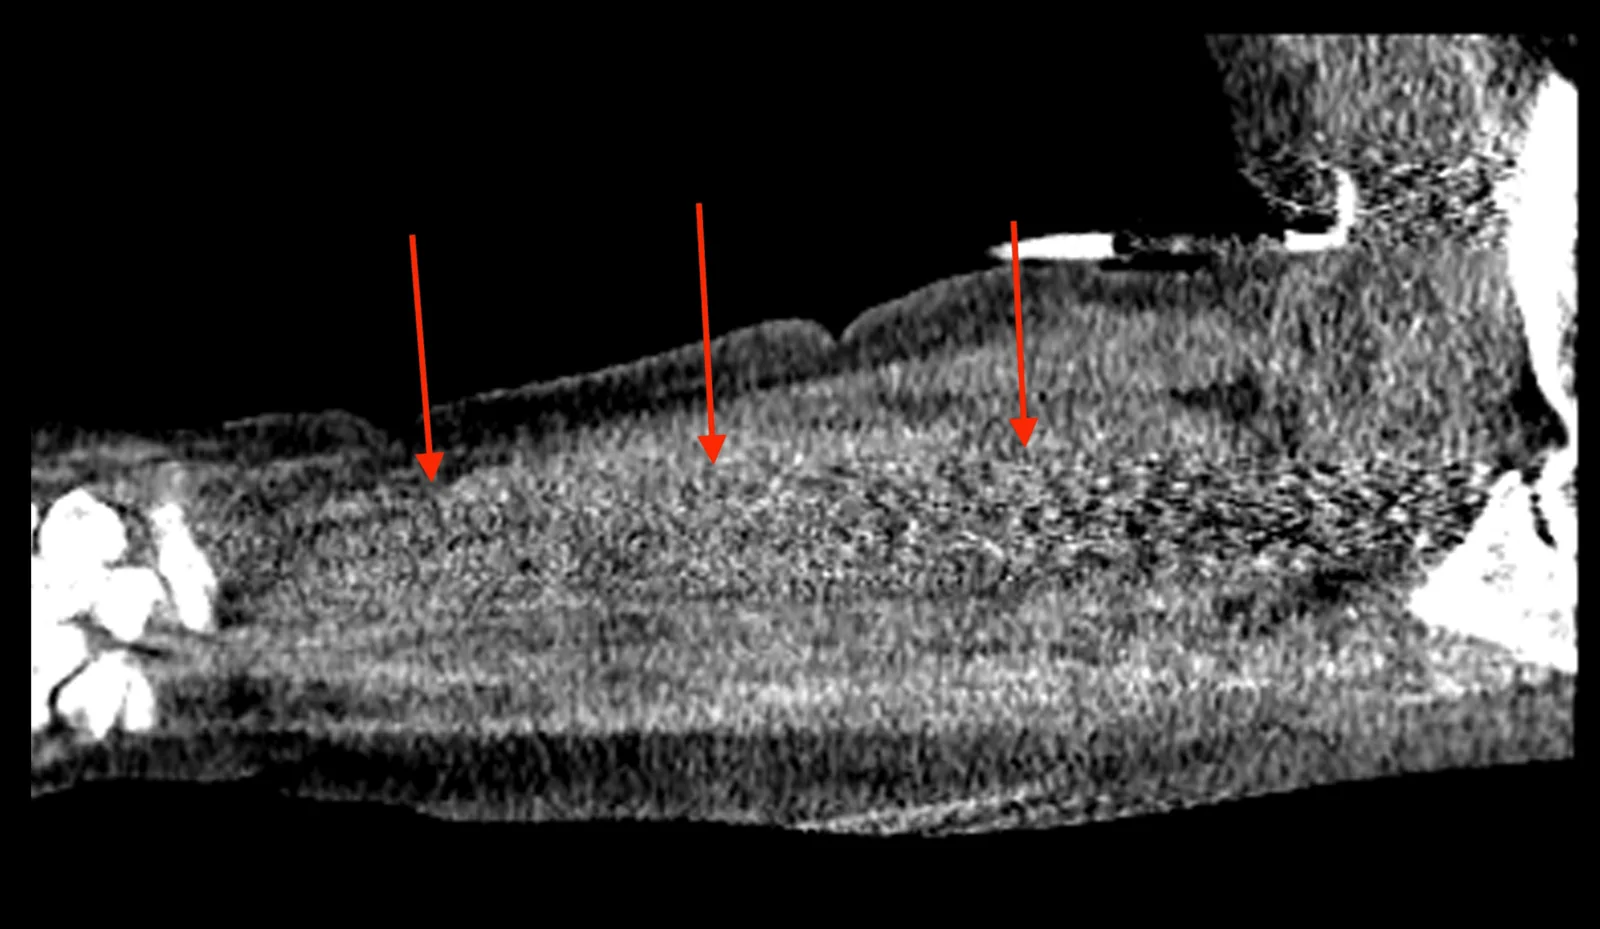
Coronal CT scan of the right forearm with evidence of severe soft tissue infection on Day 1. The red arrows highlight soft tissue swelling within the wound, supporting a diagnosis of necrotizing fasciitis. CT=computed tomography
